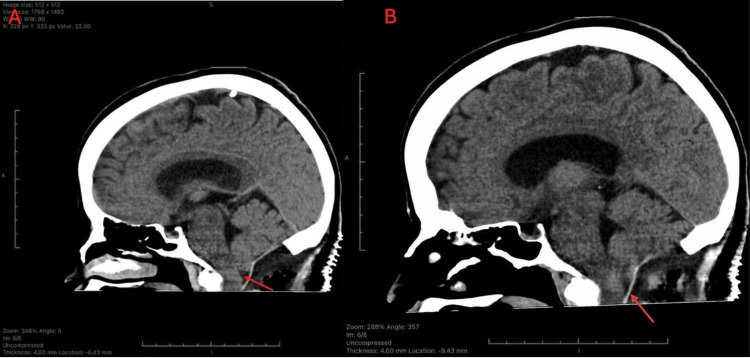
Post-operative sagittal head computed tomography (CT) following posterior fossa decompression and duraplasty. A. Demonstrates suboccipital craniectomy with bony decompression and expanded posterior fossa volume. The red arrow indicates the craniocervical junction with restored cerebrospinal fluid (CSF) spaces. B. Magnified sagittal view consistent with successful bony decompression and duraplasty without evidence of complication.
